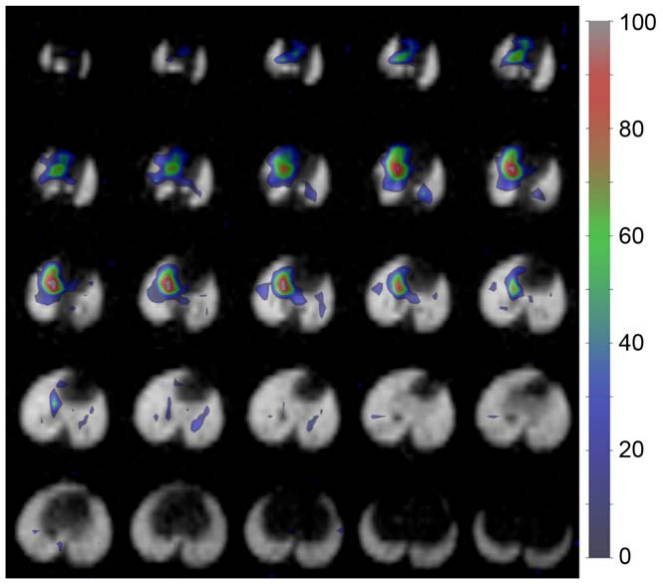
Dissolved ^129^Xe MR imaging. Axial, EC ^129^Xe infusion image (color; 2×2×2 mm^3^ nominal resolution; EC flow ∼15 ml/min) overlaid on the corresponding ventilation image (grayscale; 1×1×2 mm^3^ nominal resolution). High signal intensity is seen from the right heart and pulmonary arteries, but not from the lung parenchyma, due to RF attenuation and rapid diffusive losses of ^129^Xe from the capillary blood into the alveolar gas spaces. The signal intensity of the dissolved image (arbitrary units) is indicated in the legend.
